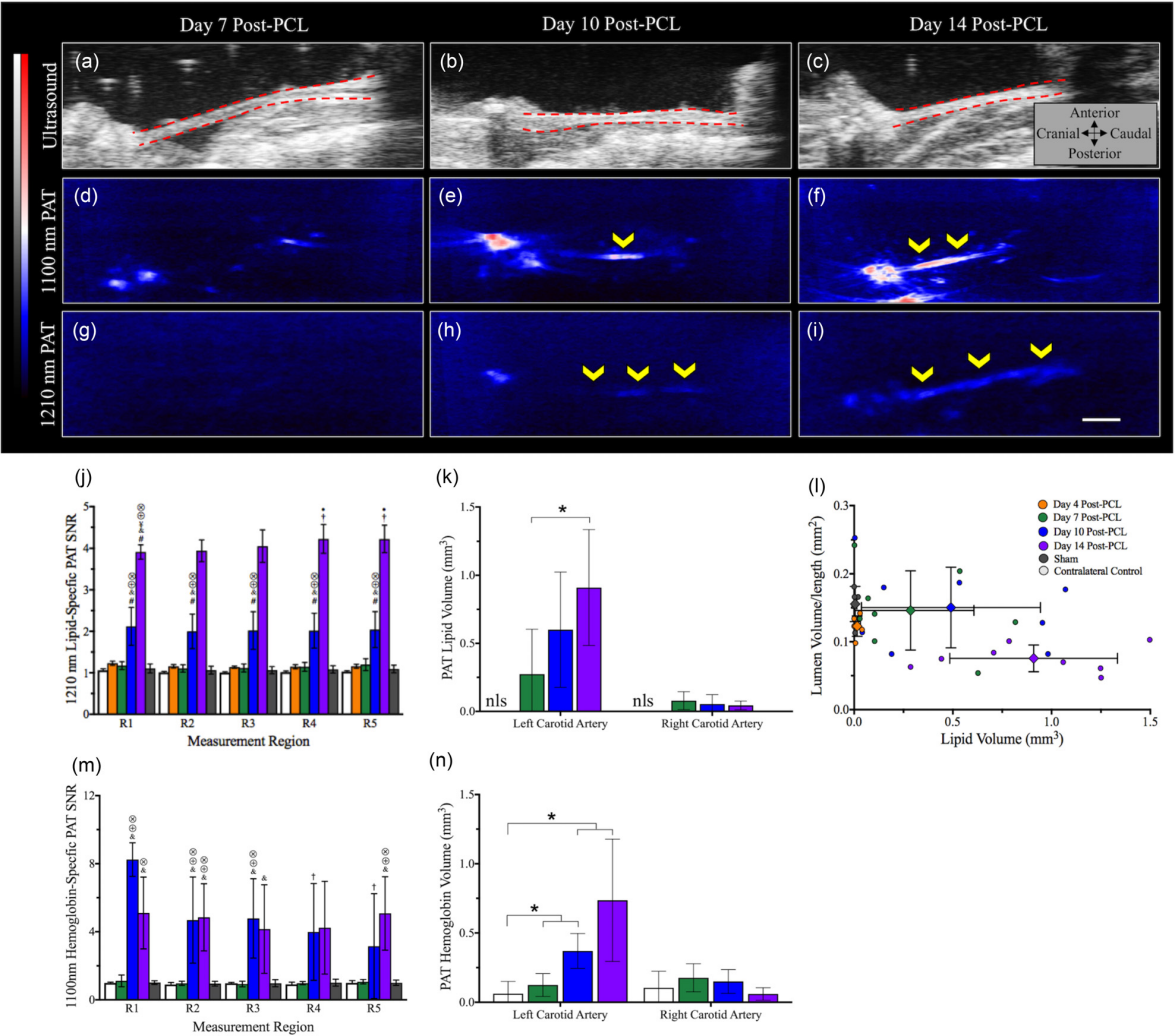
Representative *in situ* ultrasound [(a)–(c)], 1100 nm PAT [(d)–(f)], and 1210 nm PAT [(g)–(i)] images of LCA at days 7, 10, and 14 post-PCL. Regional assessment of lipid specific PAT SNR (j) and PAT lipid volume (k) suggested statistically significant and rapid lipid accumulation between days 7 and 14, with greater lipid accumulation in the caudal most regions at day 14. Comparison of the lipid volume and lumen volume/length suggests compensatory remodeling of the LCA until day 10, potentially due to Glagov remodeling, followed by a rapid decrease in the lumen volume and increase in lipid volume (l). Regional assessment of hemoglobin specific PAT SNR (m) and PAT hemoglobin volume (n) revealed the presence of intraplaque hemorrhages at day 10 with a 98.9% increase blood volume between days 10 and 14. Statistical significance determined at *p *<* *0.05 (* = significance between groups, † = significance compared to the same day in region 1, # = significance compared to day 4 in the same region, & = significance compared to day 7 in the same region, ⊗ = significance compared to sham group, ⊕ = significance compared to right contralateral control RCA). Scale bar: 1 mm.
